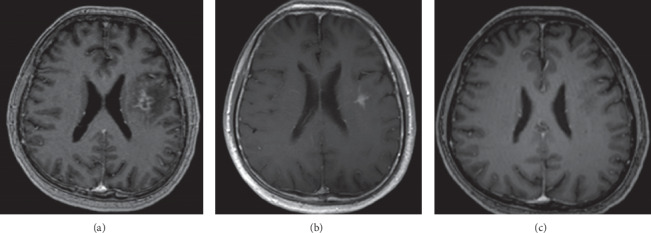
Axial FLAIR MRI images on admission, week 4 of therapy, and week 8 of therapy, respectively, showing resolution on treatment: (a) week 0, (b) week 4, and (c) week 8.
